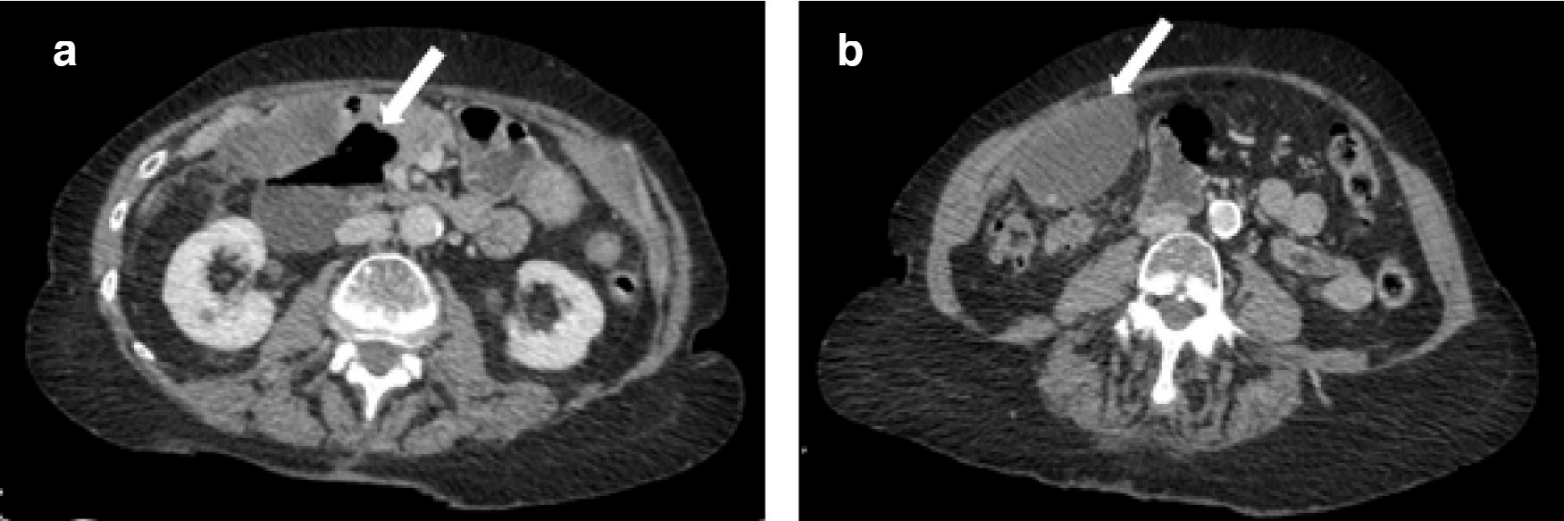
A: CT on the day of admission demonstrates a large 7.8 × 9.3 x 4.6 cm fluid- and gas-containing focus adjacent to the duodenum (arrowed) with associated fat stranding. B: The adjacent gallbladder is distended and mildly thick-walled (arrowed) and contains a calcified gallstone.
